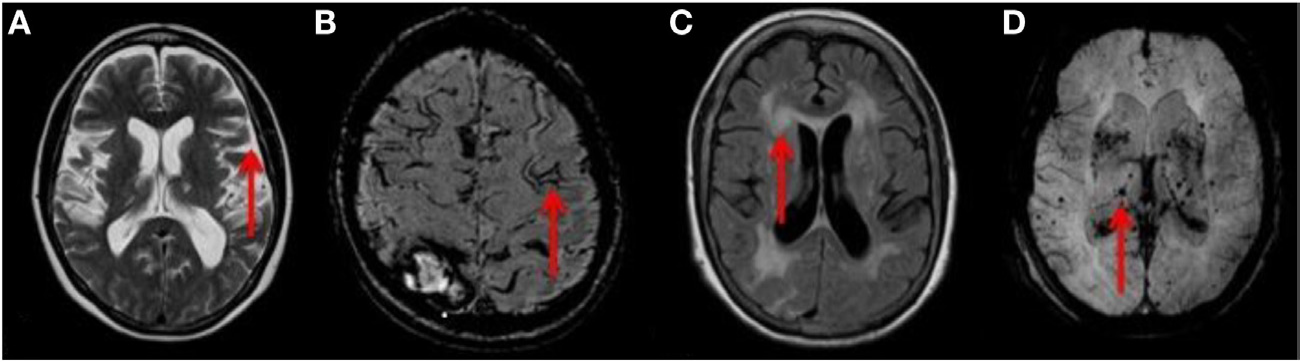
(A) T2 weighted axial MRI of the brain showing severe cerebral atrophy [Grade 4 rating using a published reference template (31)] with sulcal widening (red arrow). (B) Axial MRI of the brain demonstrating a linear gyriform hypointensity (red arrow) on SWI sequence in a cortical distribution representative of superficial siderosis. (C) T2 FLAIR weighted MRI showing large confluent lesions (red arrow) with a Fazekas 3 rating as described in the Section “Materials and Methods.” (D) SWI MIP sequence MRI of the brain showing multiple diffuse microbleeds (red arrow). Microbleeds were assessed using the validated Brain Observer Microbleed Scale (BOMBs) criteria as discussed in the Section “Materials and Methods.”
